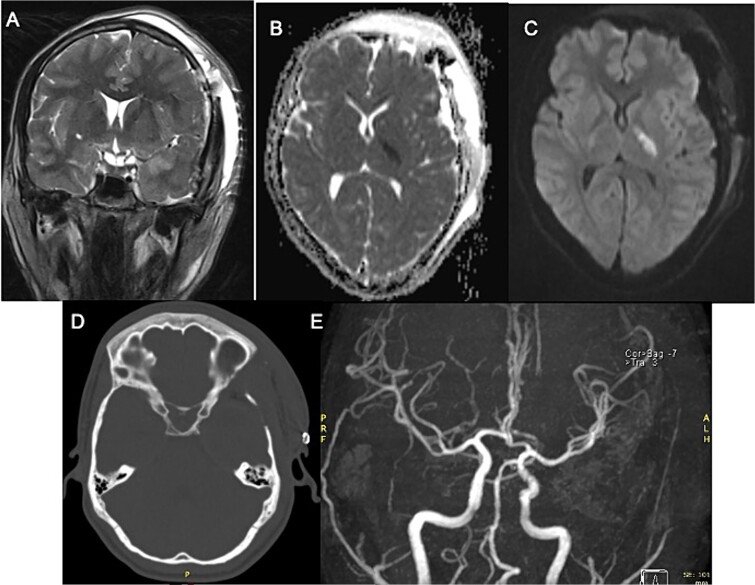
Postoperative MRI with left third cranial nerve decompression in T2-weighted sequence (A) and a high DWI signal and low ADC signal in the posterior limb of left internal capsule (B, C) with no occlusion in angiogram MRI demonstrating ischemic event (E) Head CT Scan (D) without compressive lesion.
